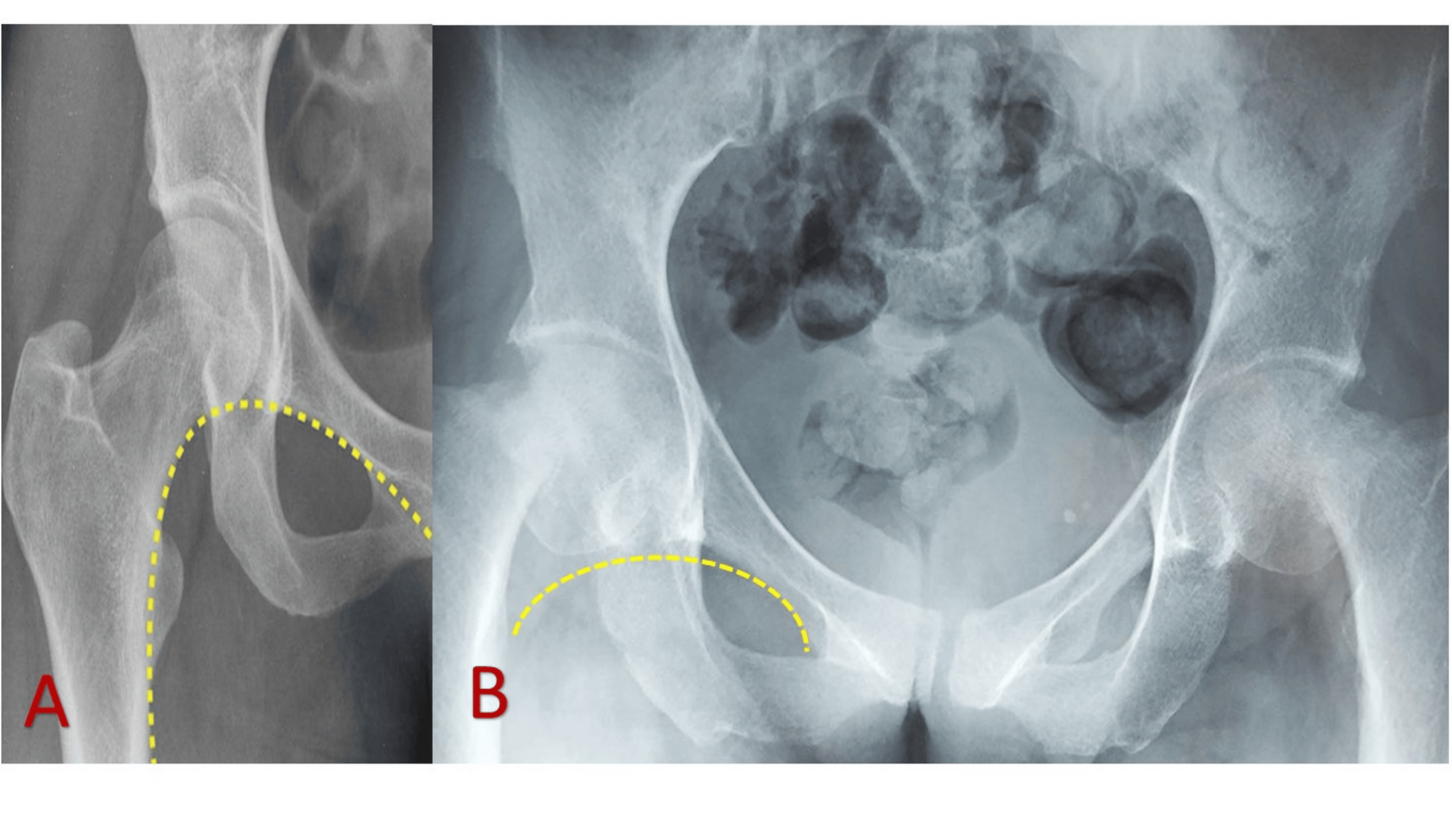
Plain X-ray of the pelvis and femur (AP view): (A) Preservation of the normal Shenton’s line with no significant findings. (B) Bilateral shortening of the femoral necks, reduced joint space, and disruption of Shenton’s line, indicative of old femoral neck fractures in a 42-year-old woman
